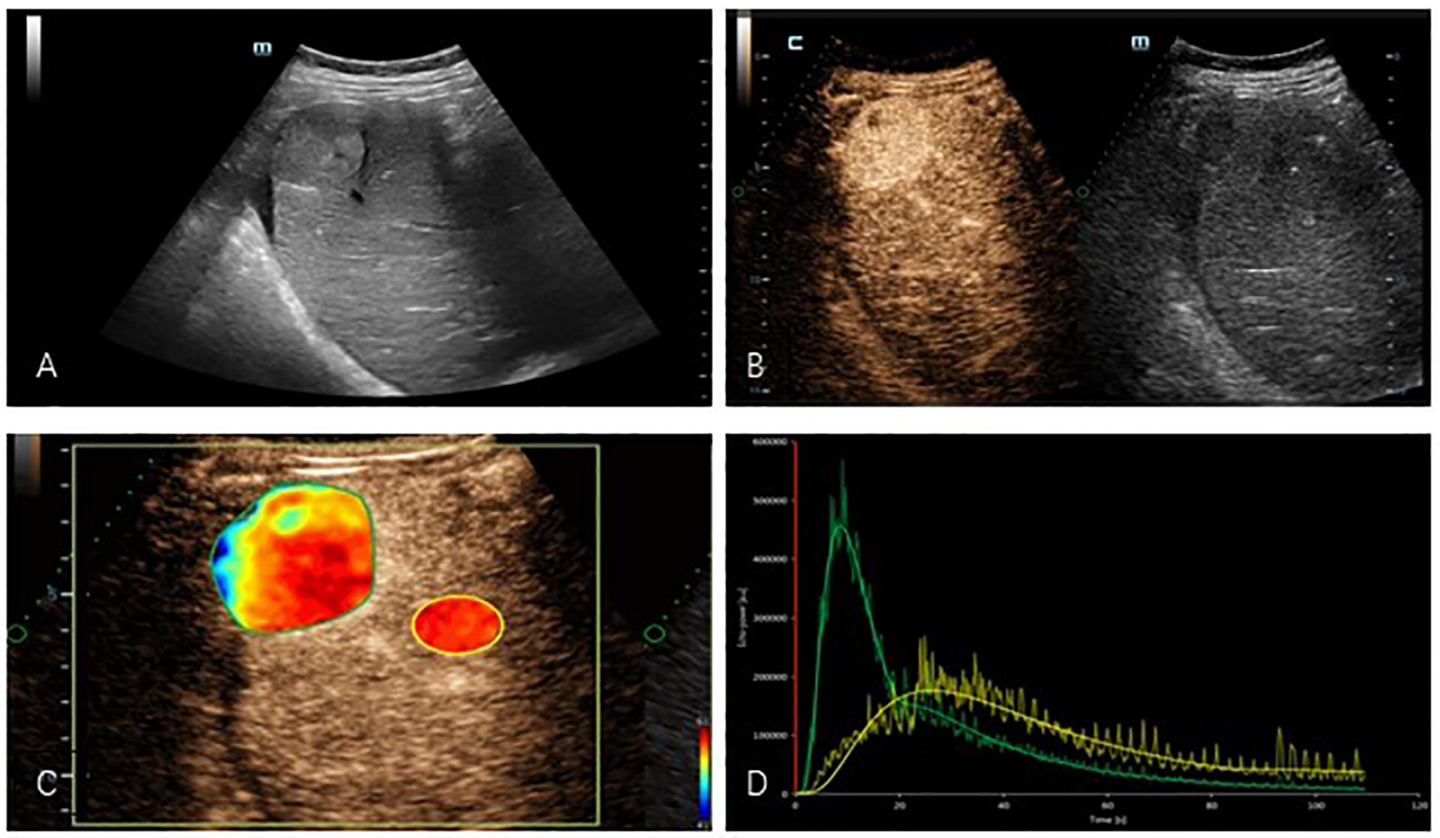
(A) Two-dimensional ultrasound showed a hyperechogenicity in the right lobe of the liver with well-defined borders and less homogeneous internal echoes. (B) Contrast-enhanced ultrasound images showed hyperenhancement in the arterial phase of the tumor. (C) Regions of interest were delineated in quantitative analysis images of masses. (D) TIC: Compared with normal liver tissue, the tumor presents a typical appearance as “fast wash-in and fast wash-out”. (yellow: normal liver tissue, green: mass).
